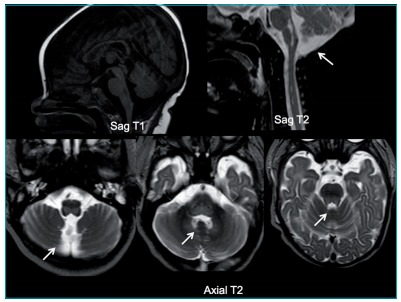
MRI: Sagittal T1, Sagittal T2 and Axial T2 views showing well-defined
